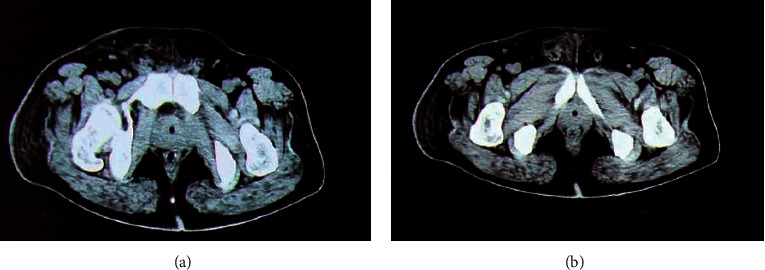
CT image with a perianal horseshoe abscess (asterisk) (case 1, a male patient, aged 53 years). (a) Axial CT image. (b) Coronal CT image.
